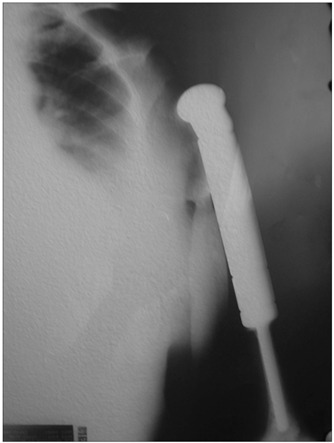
Radiograph showing wide resection of the proximal humerus with endoprosthesis replacement.
